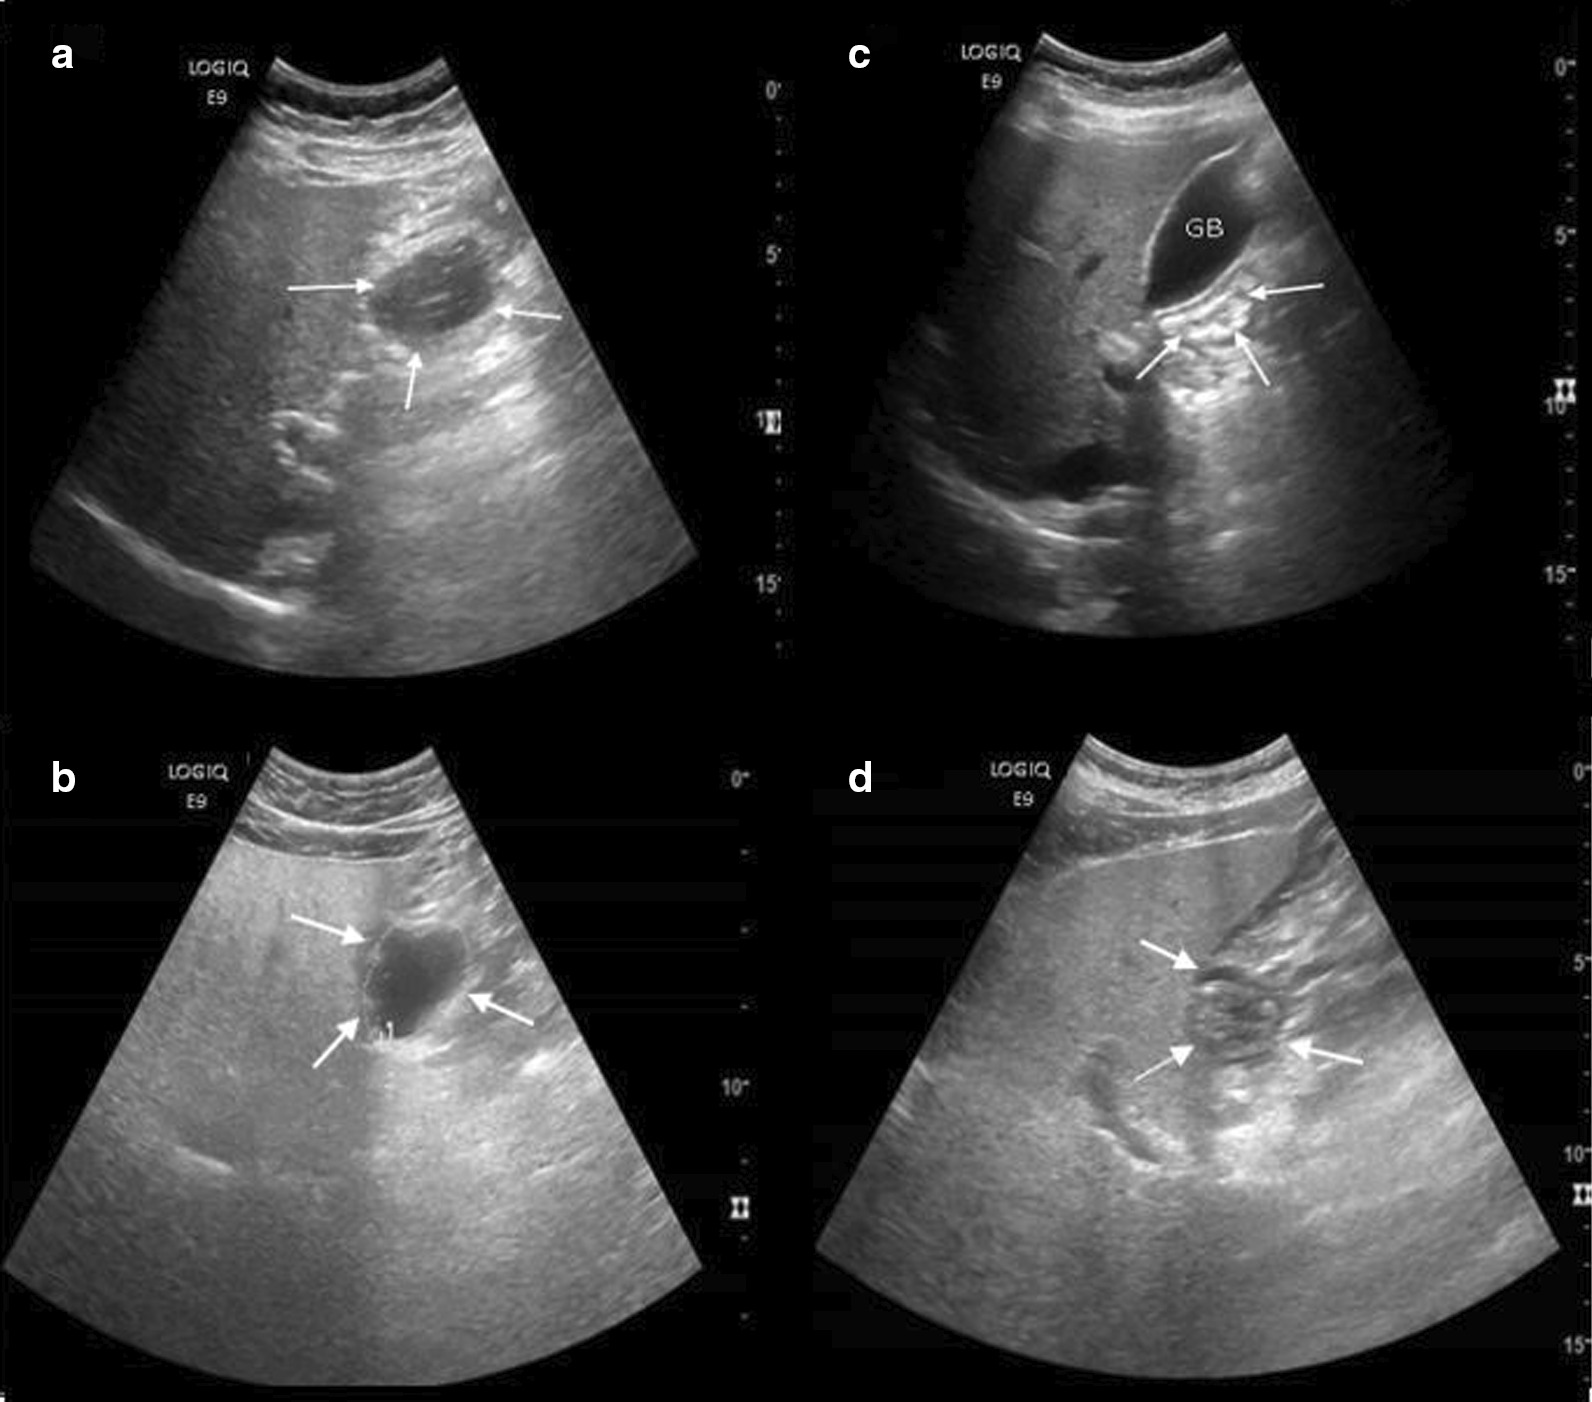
Ultrasound assessment of GRV. Cross Sectional Area of the gastric antrum (arrows) at high GRV (a, b) and low GRV (c, d) after ingestion of enteral feed. GB, gall bladder
